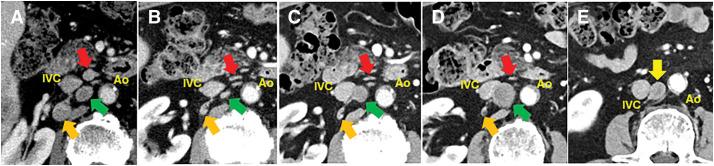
Radiological response of PALNs. (A) Pre-treatment contrast-enhanced abdominal CT showing multiple enlarged PALNs: 9 × 9 mm at the 16b1 interaortocaval region (red arrow), 15 × 10 mm at the 16b2 retrocaval region (orange arrow), and 14 × 12 mm at another 16b2 retrocaval site (green arrow). (B) After three cycles of the STRIDE regimen, the PALNs had decreased in size. (C) After six cycles, one previously regressed lymph node (green arrow) showed signs of regrowth. (D) After nine cycles, the same lymph node had enlarged to 15 × 15 mm (green arrow), whereas the other PALNs (red and orange arrows) remained reduced in size. (E) A newly enlarged 16b retrocaval lymph node measuring 15 × 12 mm appeared (yellow arrow). Ao, aorta; IVC, inferior vena cava; PALN, para-aortic lymph node
